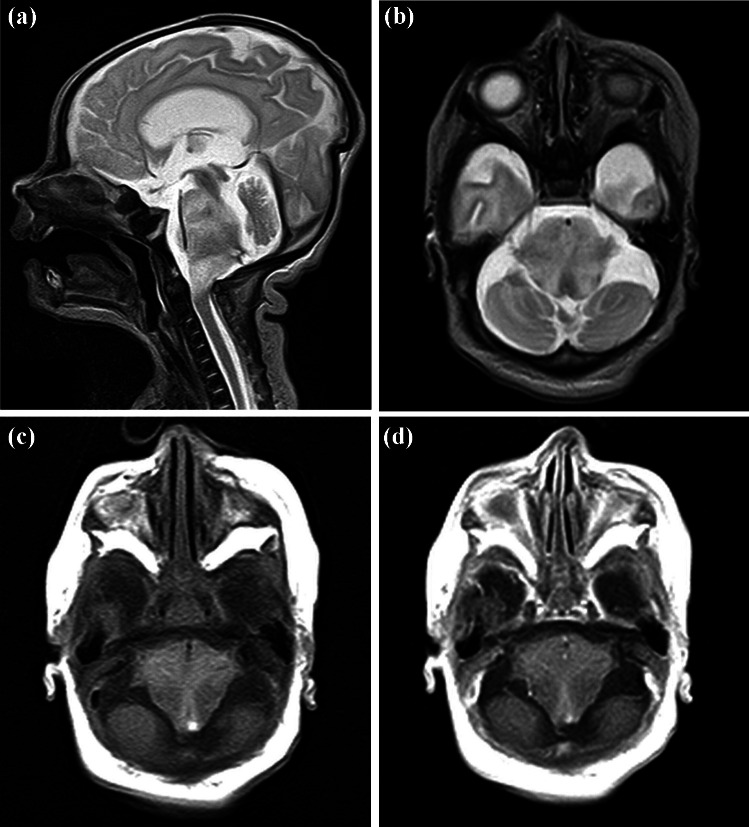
Case 4. First postnatal MRI at the twelfth day of life with sagittal (a) and axial (b) T2-weighted images demonstrating the diffuse infiltrating tumor with moderate expansion in equal parts in pons and medulla oblongata, no enhancement after application of gadolinium-based contrast agent (T1-weighted images without (c) and with contrast agent (d), both axial planes)
